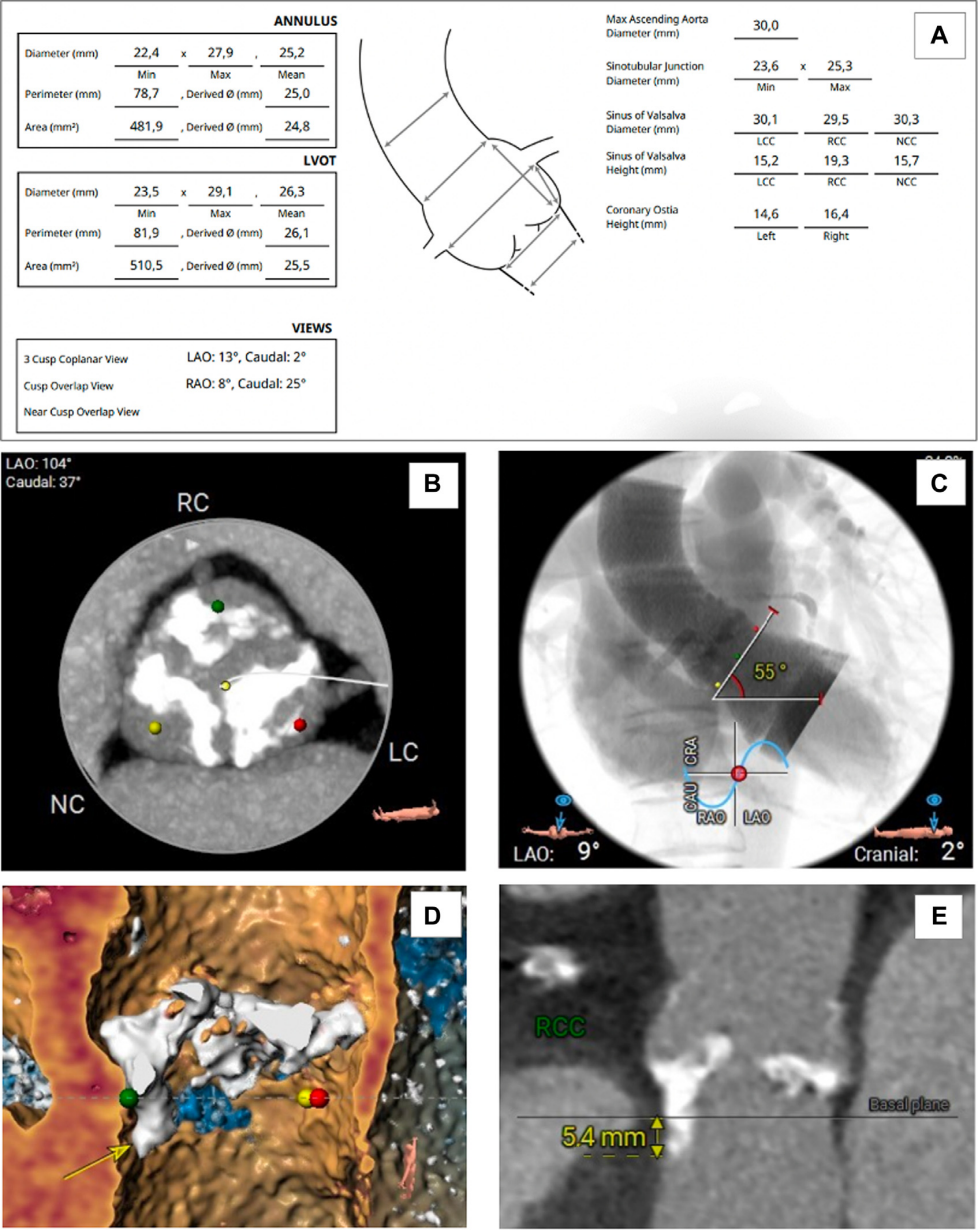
Cardiac and Aortic Computed Tomography (A) Measurements of the aortic annulus, left ventricular outflow tract, and aorta. (B) Heavily calcified aortic valve. (C) Aortic valve angulation relative to the horizontal plane. (D and E) Calcification in the left ventricular outflow tract (yellow arrow). CAU = caudal; CRA = cranial; LAO = left anterior oblique; LC = left coronary; LVOT = left ventricular outflow tract; Max = maximum; NC = noncoronary; RAO = right anterior oblique; RC = right coronary; RCC = right coronary cusp.
